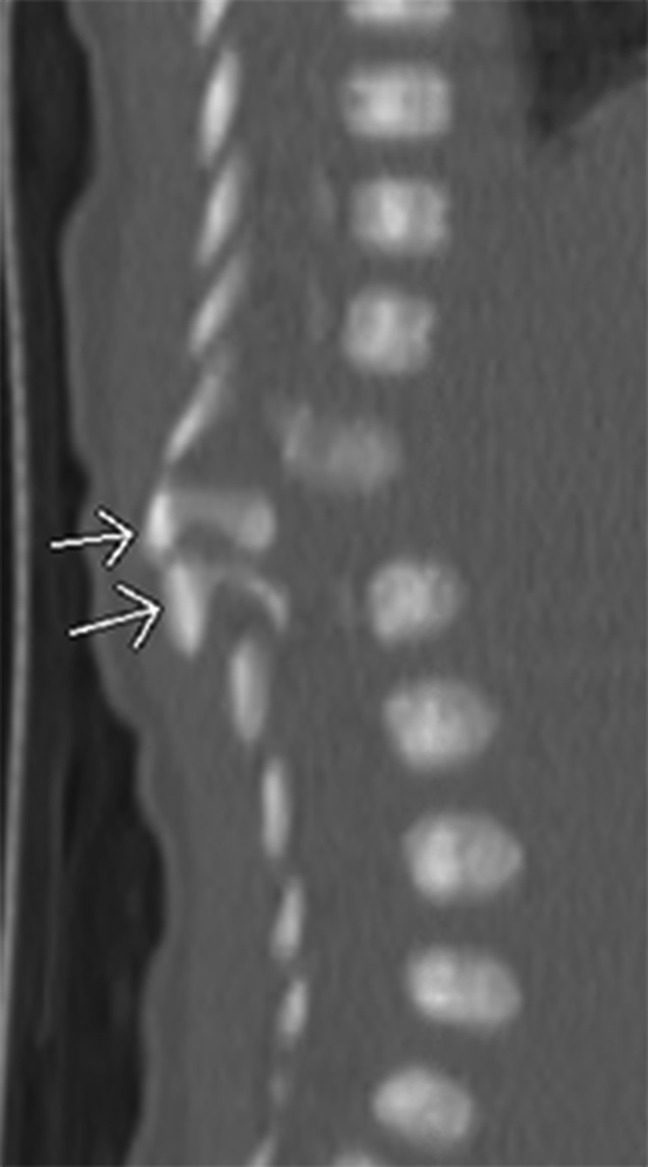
Sagittal CT scan taken at day 2 of life (at an outside institution) demonstrates two posterior hemivertebrae, with osseous posterior elements visualized without a corresponding vertebral body anteriorly. (Reproduced with permission from the Children's Orthopaedic Center, Los Angeles, CA.)
